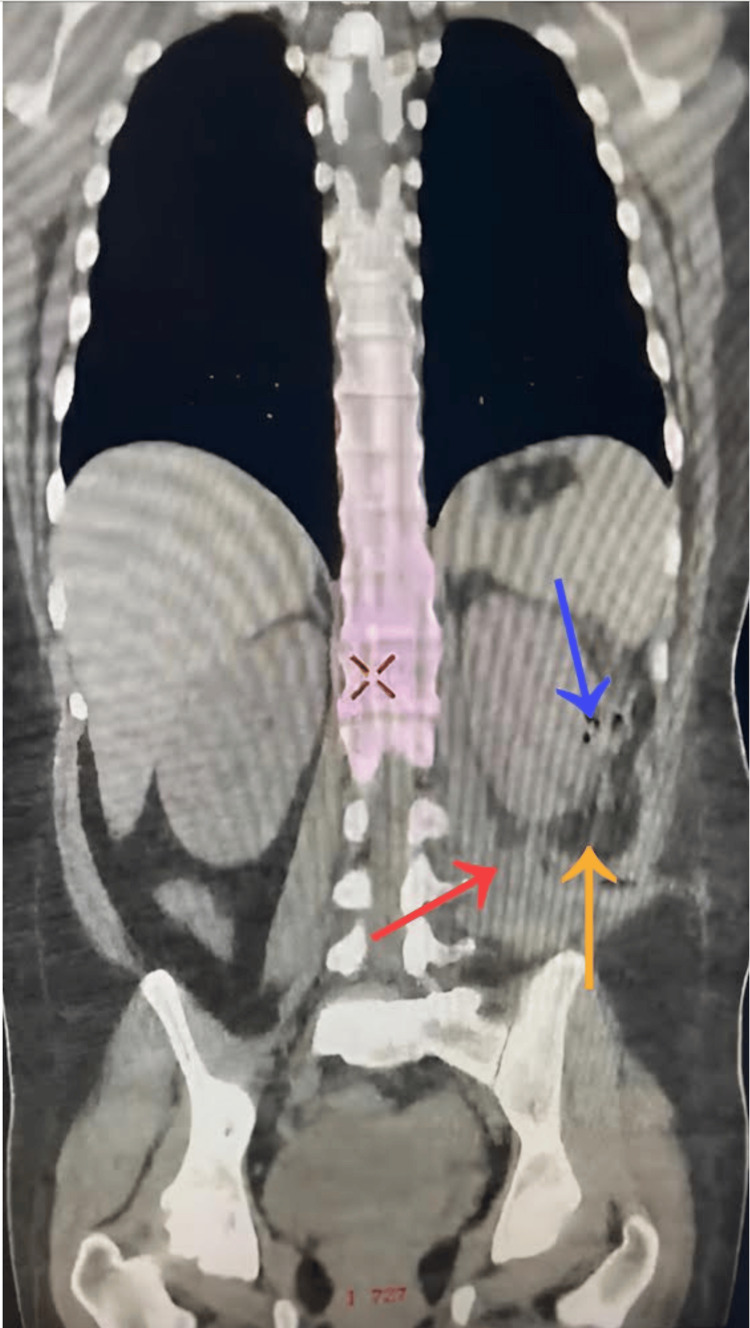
Post-surgical CT revealing residual abscess Fluid accumulation (red arrow) is seen in the left renal fossa, accompanied by emphysema (blue arrow) and a slight amount of striated adipose tissue (yellow arrow).
